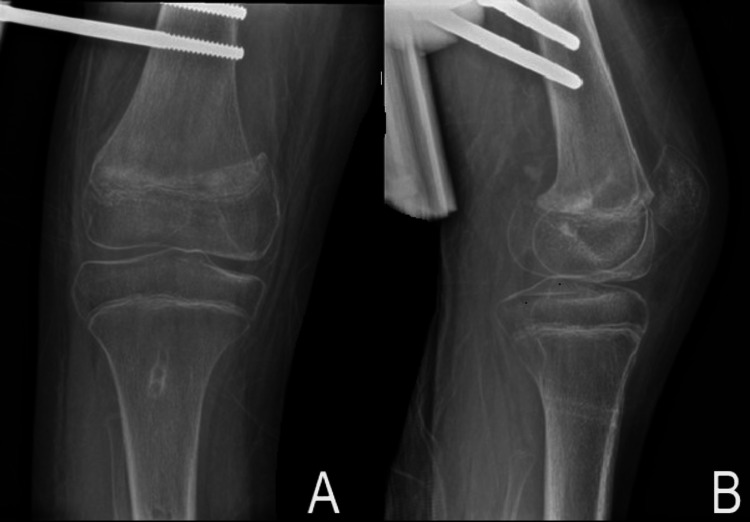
Radiographs of the right knee Anteroposterior (AP) (A) and lateral (B) radiograph of the right knee displaying type I Salter-Harris fracture over the distal femur.
